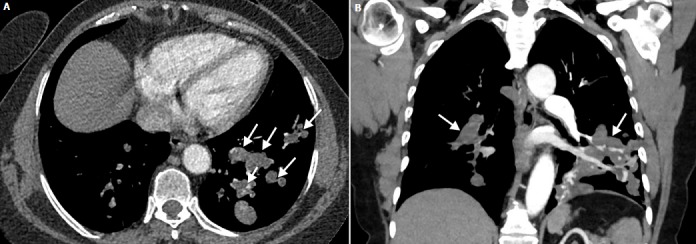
Extraluminal extension of the tumor (A) and grape like shape of involved segmental and subsegmental branches of PA (white arrows) are observed on axial (A) and coronal reconstructed (B) CT images
